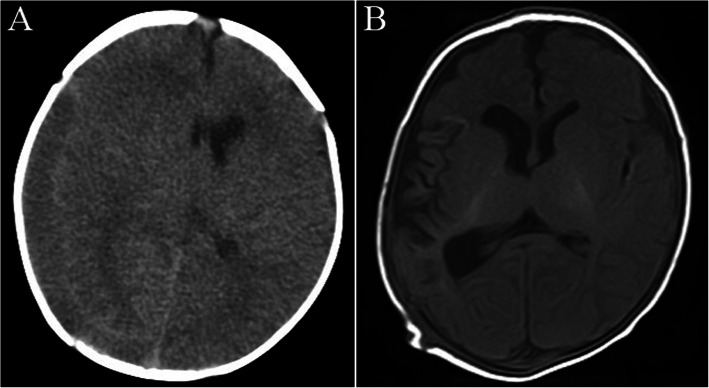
The change in imaging of the head during hospitalization. CT scan displayed a right parietal temporal hematoma with a midline shift (a). After subdural hematoma removal and external drainage of subdural hemorrhage, MRI revealed atrophy of the right cerebral hemisphere with cortical necrosis and mild dilatation of the third ventricle. No detection of intraventricular hemorrhage was found (b)
